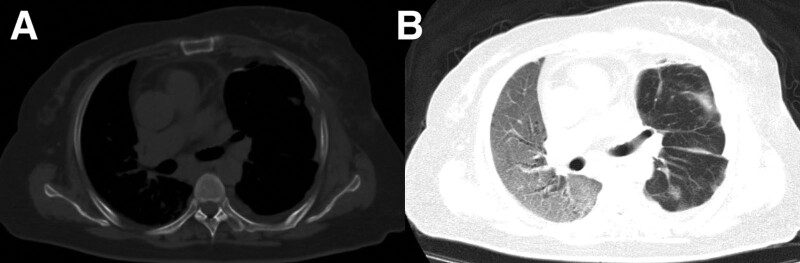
CT imagines of the patient obtained 5 d after presentation to the ICU shows diffuse infiltration of the right lung. CT = computed tomography, ICU = intensive care unit.
